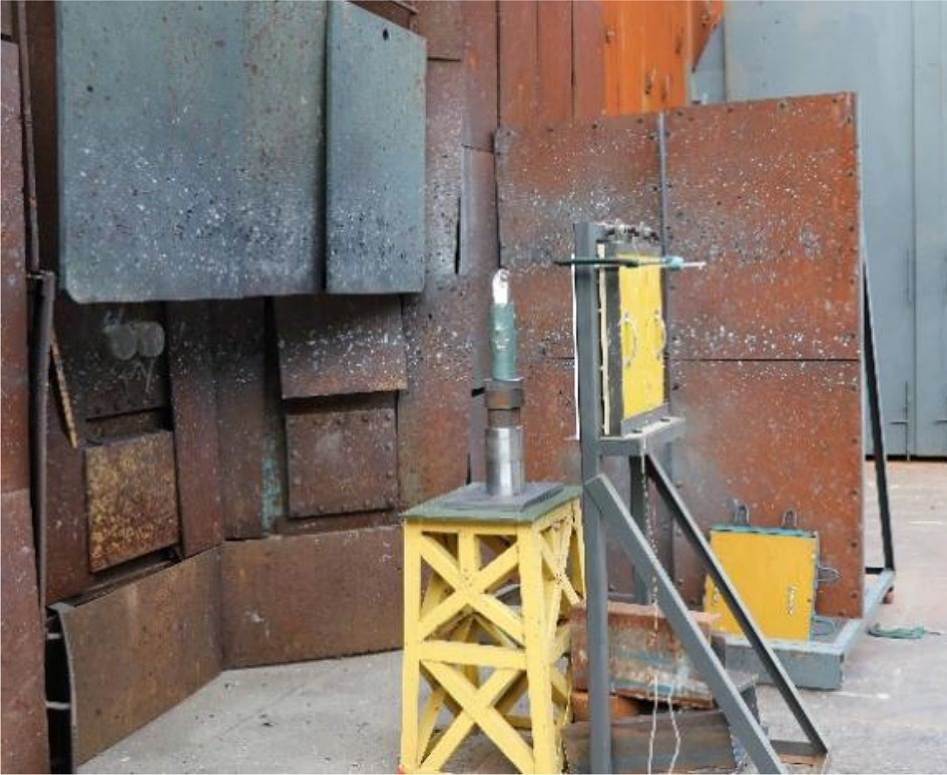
Setting of pulse X-ray experiment.
